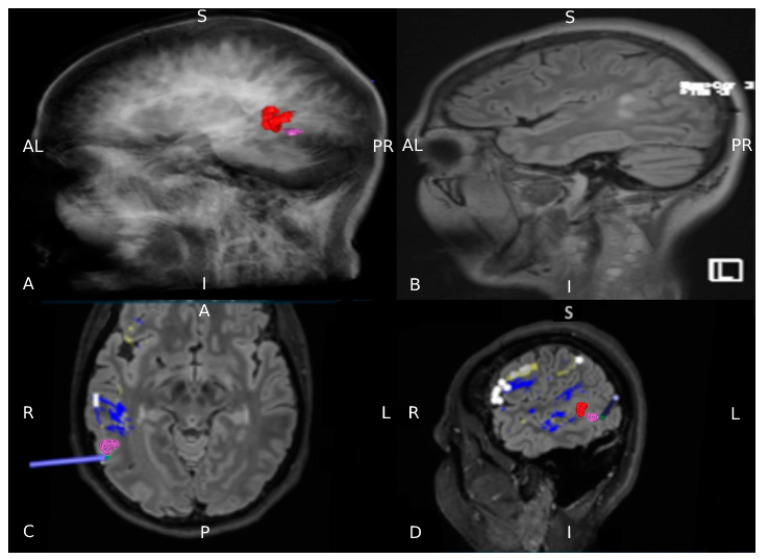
rnTMS-based planning using StealthViz software. (A) The anterior and eloquent part of the lesion identified by nTMS is colored in red and the posterior non-eloquent part in violet, (B) 3D FLAIR anatomical images for comparison. (C,D) A subtotal resection was planned and guided by neuronavigation (in violet the non-eloquent part).
